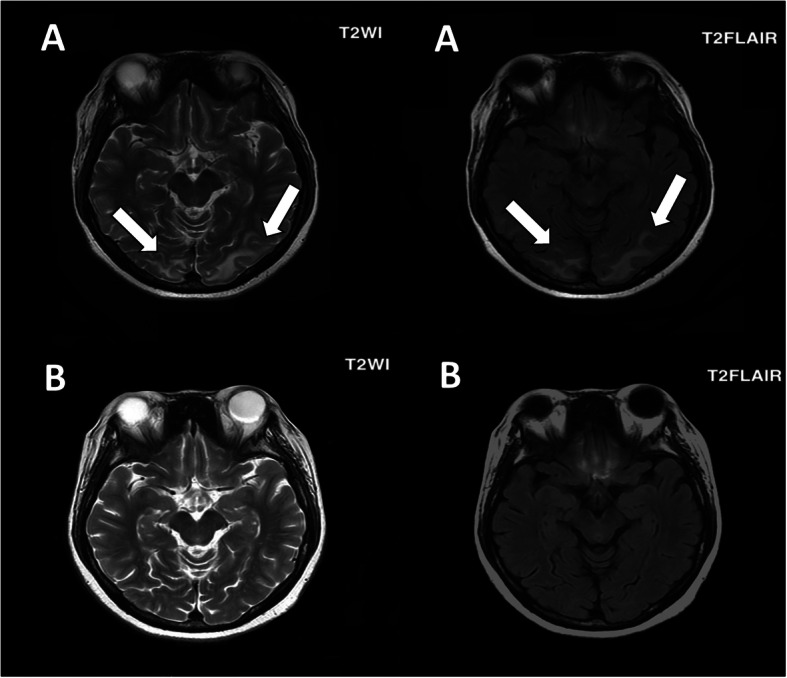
a High signal intensity on magnetic resonance (MR), T2-weighted imaging and MR T2-weighted fluid-attenuated inversion recovery imaging in the parietal–occipital lobes observed 2 days after the seizure episodes. b The lesions had disappeared 3 months later
